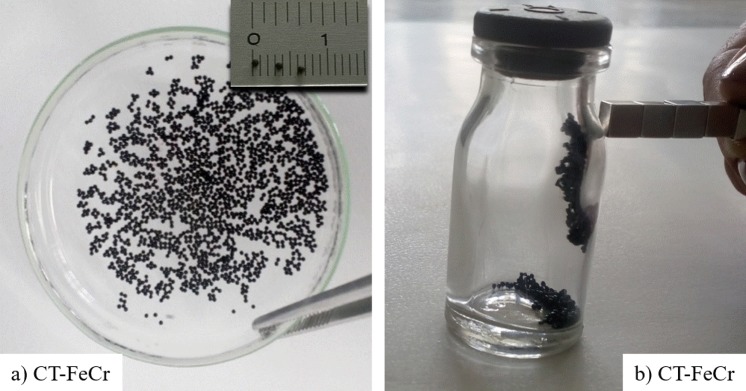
Photographic images of CT-FeCr beads (a) beads, and a dry bead of 1.0 mm diameter is shown in the insert, (b) magnetism.
